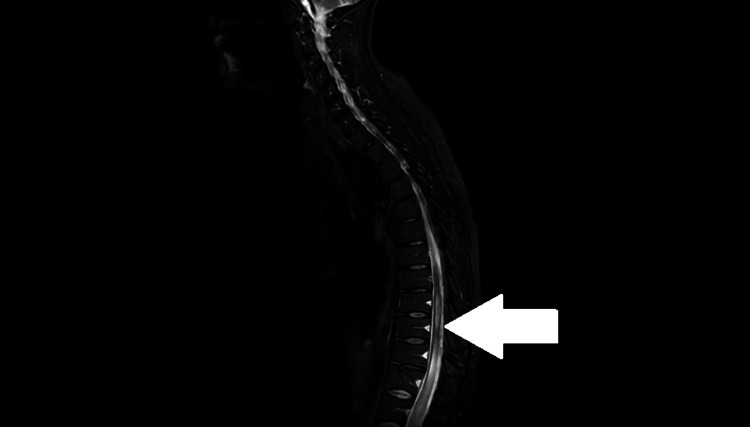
MRI spine T2-STIR sagittal sequence showing a hyperintense lesion from T9 to conus medullaris (White arrow) consistent with LETM. Sagittal T2-weighted and STIR sequences MRI of the thoracic spine demonstrates a longitudinally extensive hyperintense lesion extending from T9 to the conus medullaris (indicated by the white arrow). This imaging pattern is characteristic of longitudinally extensive transverse myelitis (LETM), a hallmark feature of NMOSD, and helps differentiate it from lupus myelitis, which typically involves shorter cord segments.
